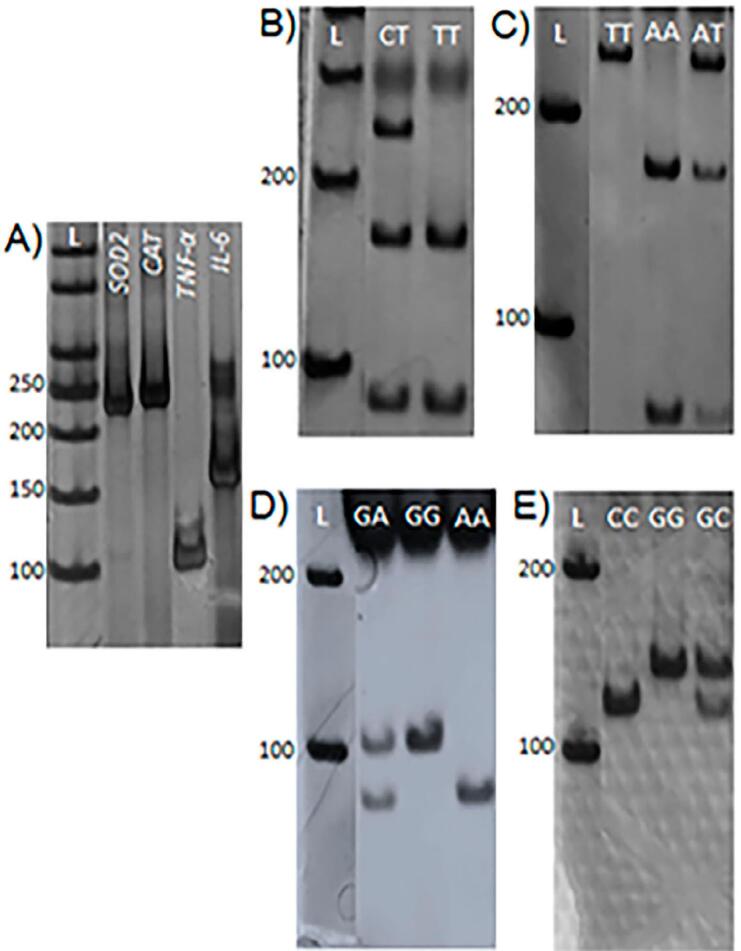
Representative fragments of PCR-RFLP reactions for each of the studied SNPs. A) Amplified fragments for each SNP: L – DNA ladder; *SOD* 2 (246 bp); *CAT* (249 bp); *TNF-* α (107 bp); *IL-6* (156 bp). B) *SOD* 2 restriction fragments: CT (246, 157, 89 bp) and TT (157, 89 bp); C) *CAT* restriction fragments: TT (249 bp), AA (175, 74 bp) and AT (249, 175, 74 bp). D) *TNF-* α restriction fragments: GA (107, 87 bp), GG (107bp) and AA (87 bp). E) *IL-6* restriction fragments: CC (117bp), GG (139bp), GC (139, 117bp)
